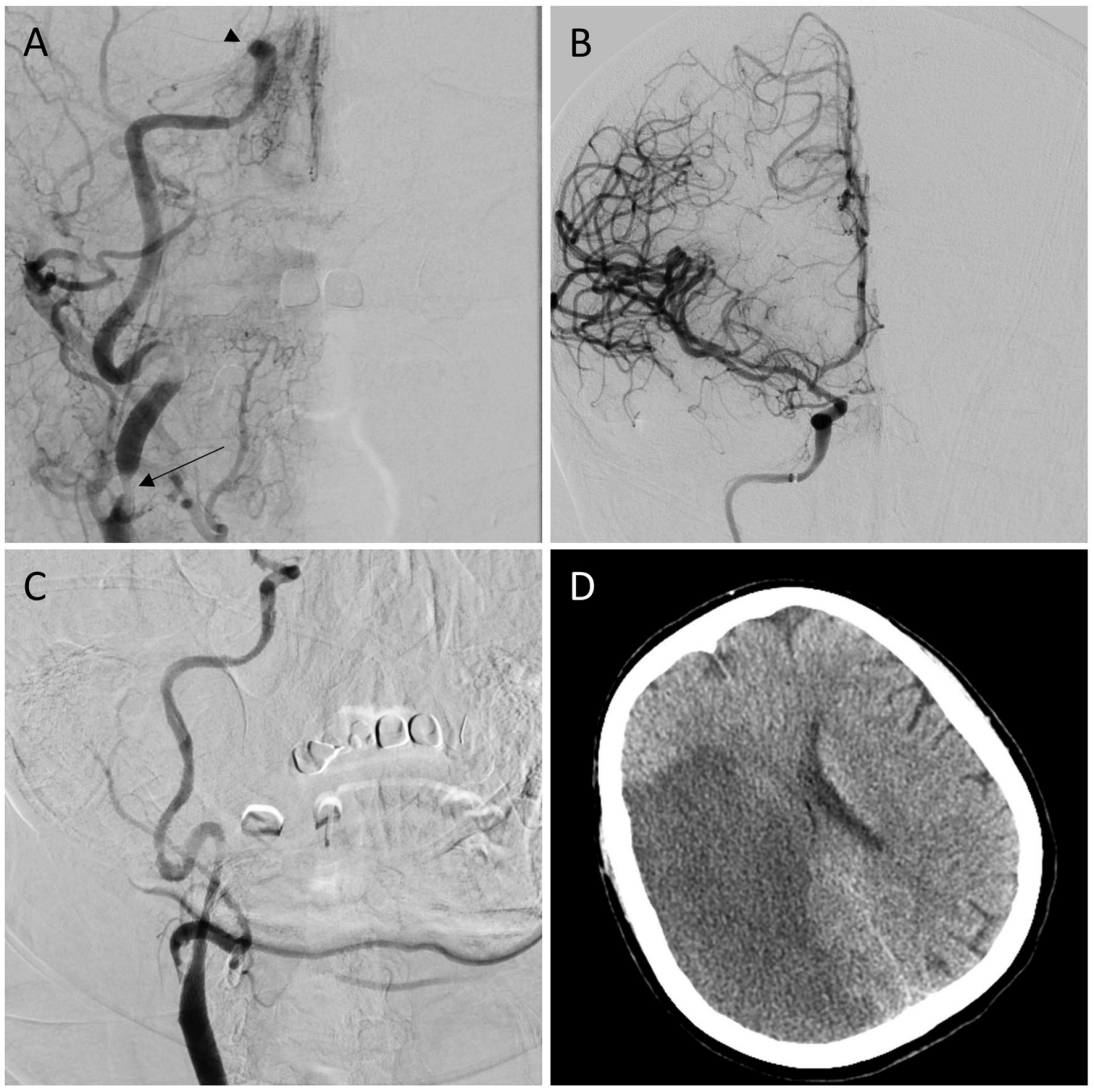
(A) Anterior-posterior (A–P) projection of cerebral angiogram shows the right internal carotid artery terminus occlusion (arrowhead) with stenosis in the cervical portion (arrow). (B) A–P projection of cerebral angiogram after mechanical thrombectomy shows Complete recanalization (mTICI 3). (C) A–P projection after carotid artery stenting shows successful angioplasty and stenting of cervical carotid artery stenosis. (D) Corresponding CT brain shows cerebral infarction at the right middle cerebral artery territory.
